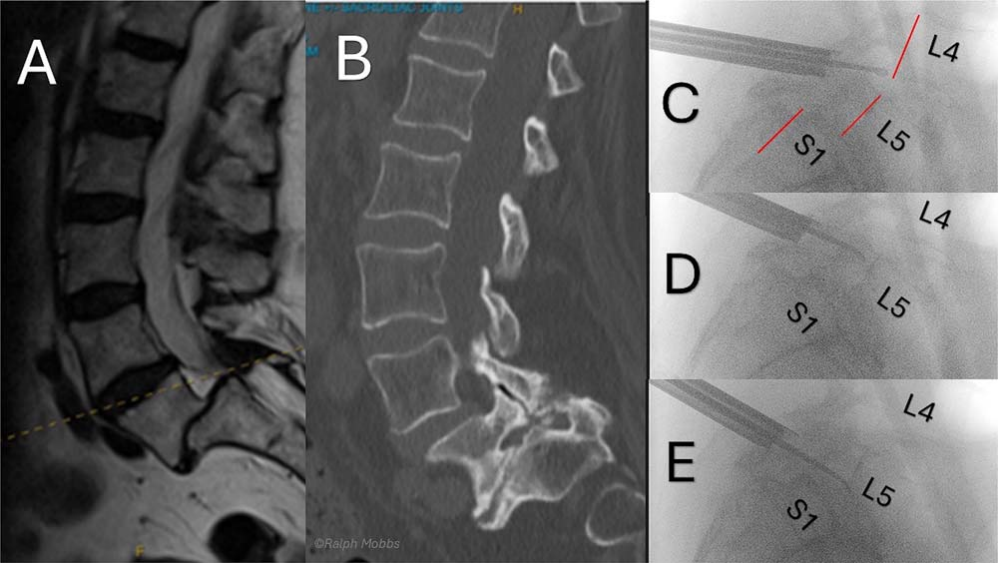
Preoperative and intraoperative imaging of case 3. (A) Sagittal T2-weigthed MRI demonstrating grade II+ degenerative spondylolisthesis at the L5/S1 level. (B) Sagittal CT demonstrating severe foraminal stenosis at L5/S1. (C–E) Intraoperative fluoroscopic images showing placement of the working channel and guidewire using forceps for targeted decompression.
